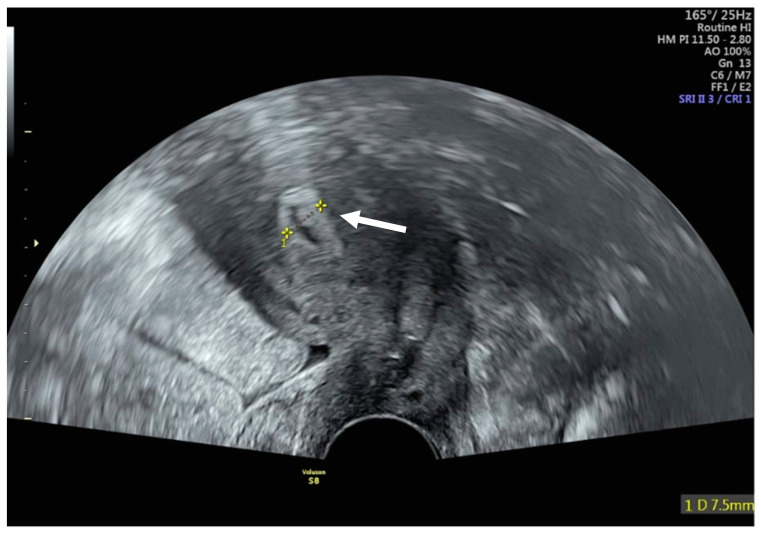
Transvaginal ultrasound demonstrating preserved endometrial function. Transvaginal ultrasound performed in the follicular phase shows an endometrial thickness of 7.5 mm (white arrow), consistent with preserved endometrial function.
